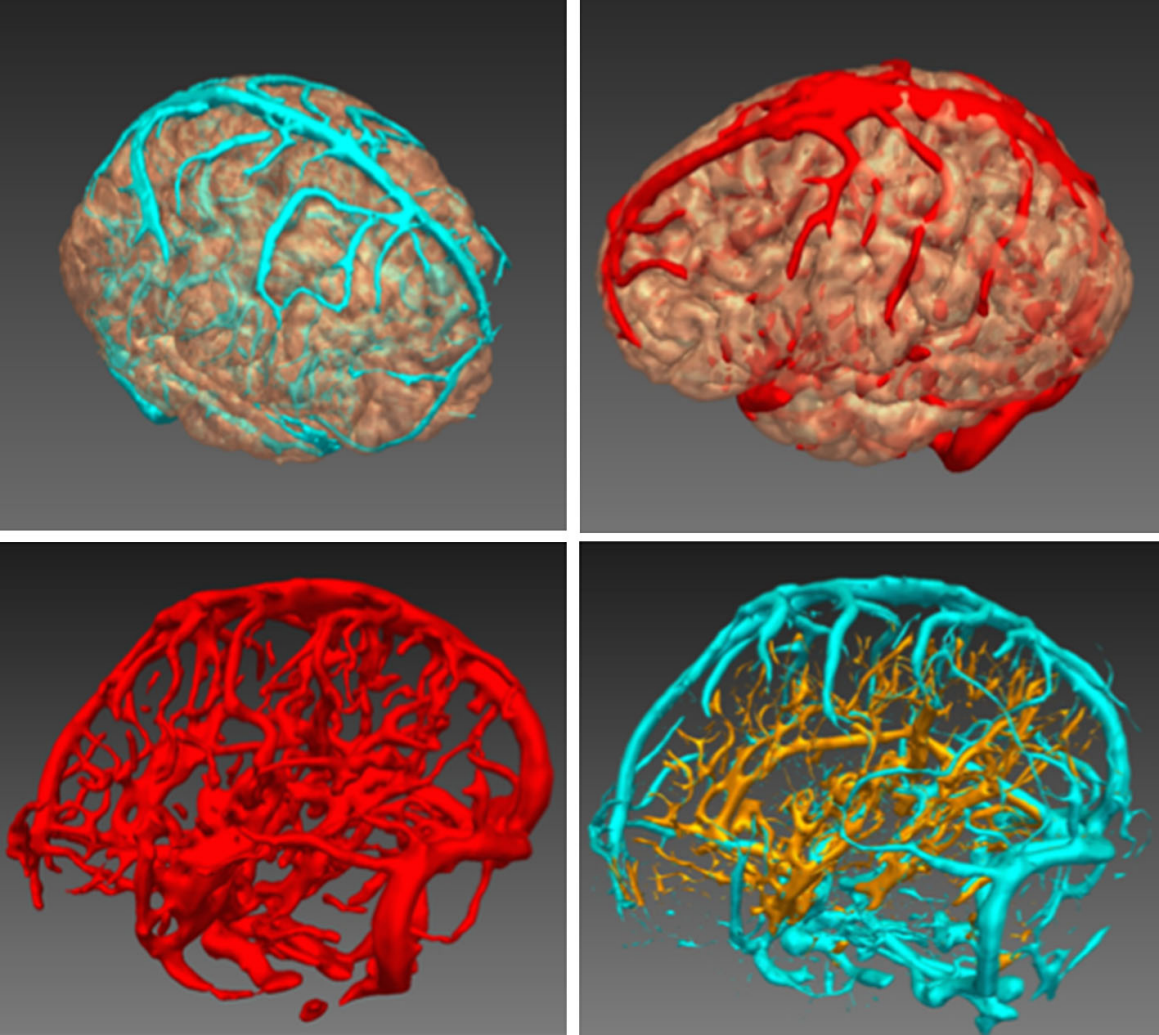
Integration into the computer-assisted planning system. On *top*, examples of displayed extracted vessels using different *colour schemes*. On *bottom left*, display of a segmented vascular tree contrasted with the combined single-modality segmentation, *right*, from 3DPC (*blue*) and CTA (*gold*). The results obtained with the proposed method contain less noise
